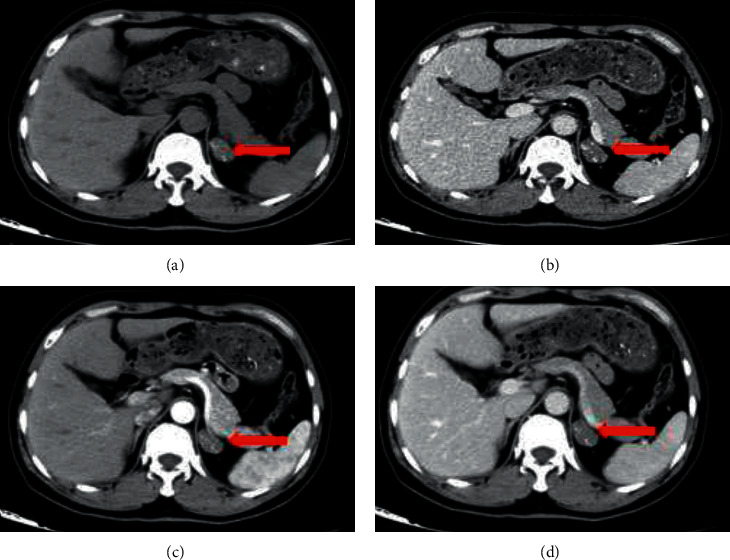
Left adrenal cavernous hemangioma with a tumor size of 2 cm. CT imaging showing an oval, well-defined, obvious calcified mass with mild enhancement (red arrow) in the left adrenal region. (a) CT unenhanced. (b) Arterial phase of CT. (c) Venous phase of CT. (d) Delayed phase of CT.
